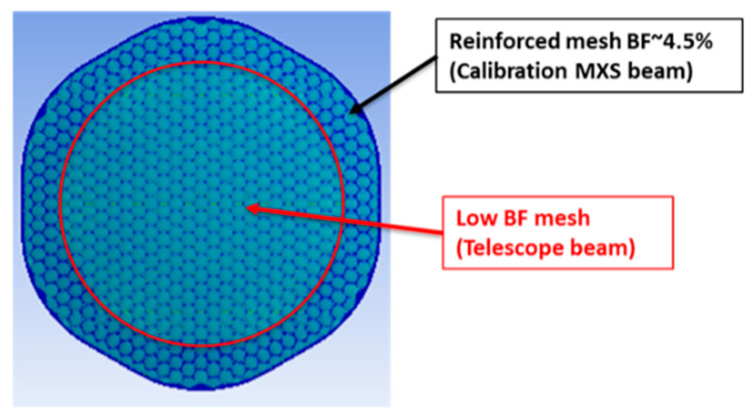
Graphical representation of the optimized mesh design used on the model D. The mesh has wider mesh bars in the outer ring illuminated only by the calibration modulated X-ray sources.
